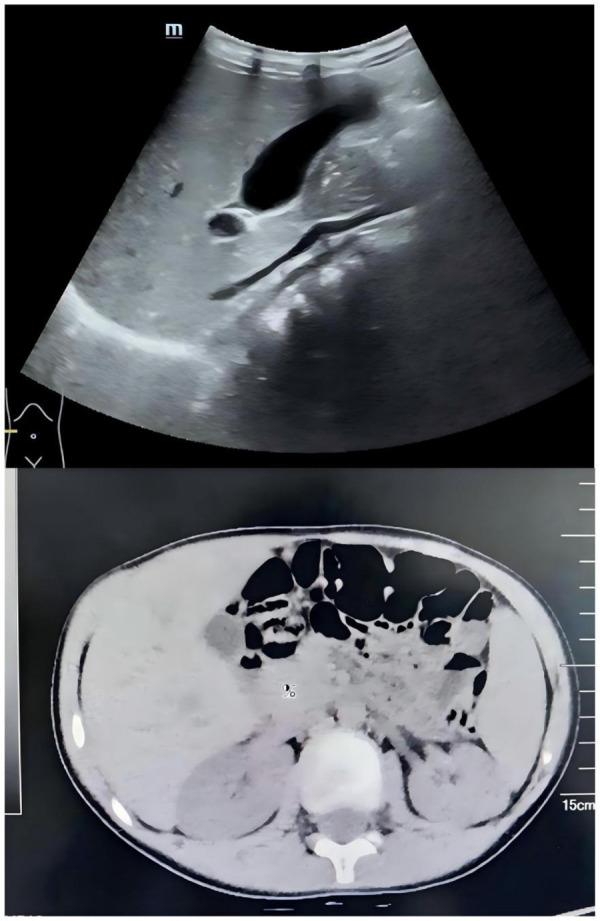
Follow-up abdominal ultrasound findings during recovery (June 6, 2025). Follow-up imaging demonstrated near-complete resolution of gallbladder wall thickening and improvement in splenomegaly, in parallel with clinical recovery.
